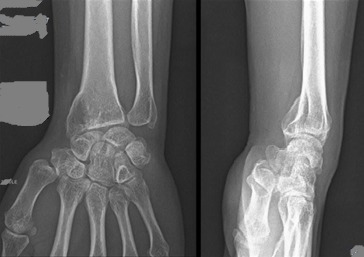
Radiographs obtained 2 years later showing an anatomical position of the radial head and the fracture was healed in good alignment
